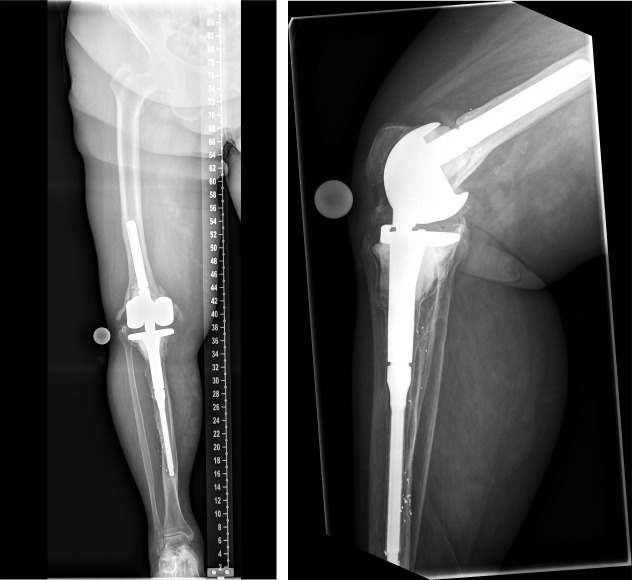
Preoperative X-ray of the right knee joint (in 2016) showing aseptic loosening of the components
